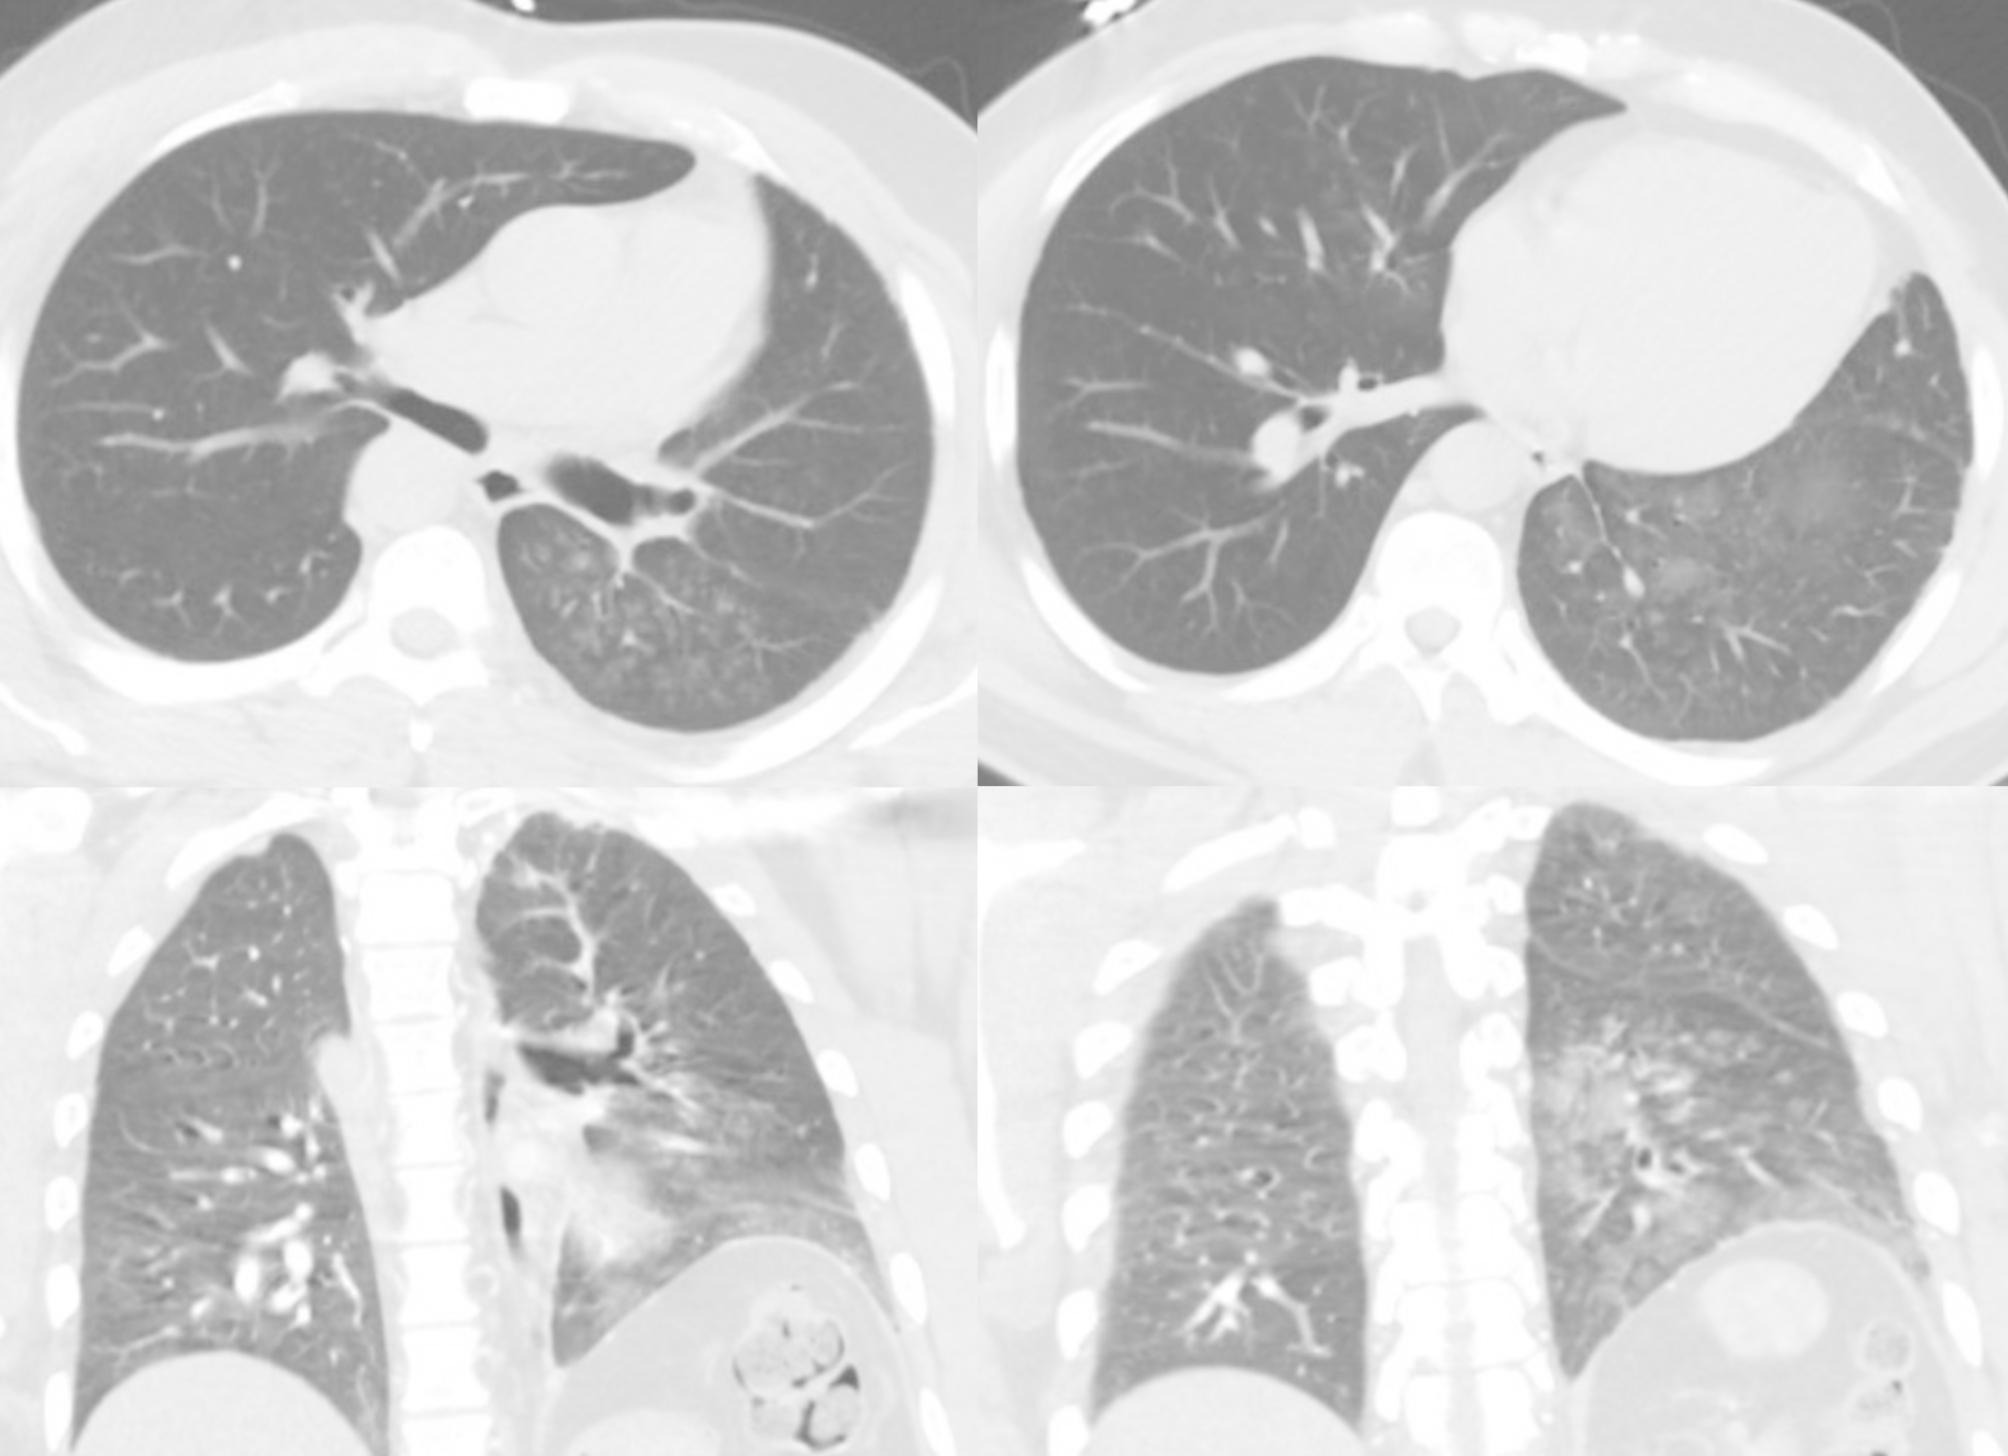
Pre-operative computed tomography of lung parenchyma in axial and coronal views
